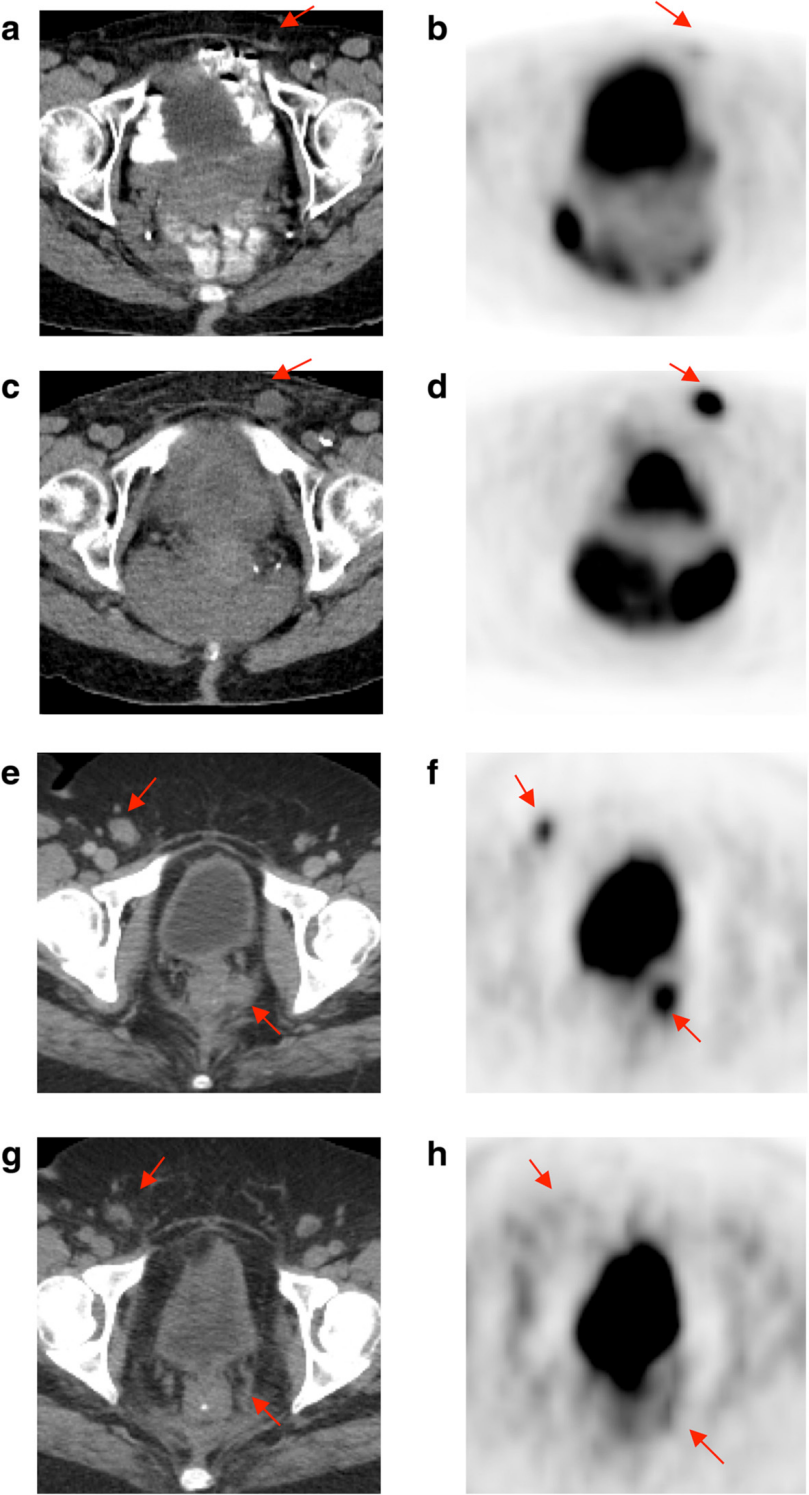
Representative^18^F-FDG-PET/CT images showing progression in a patient without an exon 11 KIT mutation (a-d) and response in a patient with an exon 11 KIT mutation (e-h) at baseline (a-b, e-f) and after 1 month of therapy (c-d, g-h). Red arrows point to sites of disease. Reprinted with permission. © (2013) American Society of Clinical Oncology. All rights reserved. From: Hodi S, et al. J Clin Oncol. 31(26), 2013:3182-3190.
